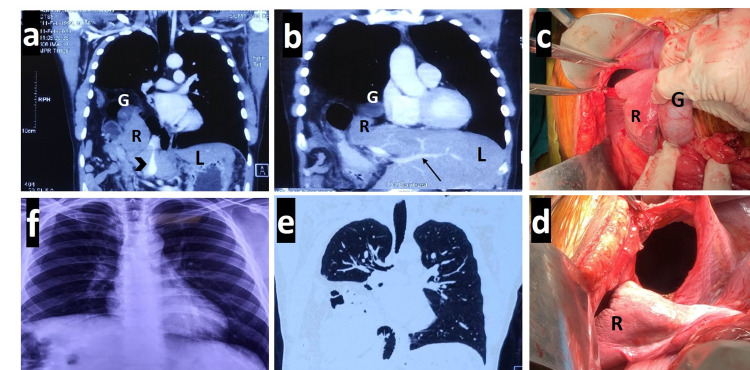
Clockwise from upper left: (a) Coronal contrast CT section of patient 1 showing multiple abdominal viscera entering the right thorax, namely, the colon and small bowel, gallbladder (marked G), and atrophied right liver (marked R). The hypertrophied left liver (marked L) and the middle hepatic vein (marked with black arrowhead) are shown. The right hepatic vein is probably obliterated. (b) Another coronal CT image depicting the complete extent of left liver hypertrophy with the rotated left portal vein axis (marked with black arrow). (c) and (d) Operative images showing the well-defined right posterolateral defect after reduction of contents, with the atrophied right liver (R) and the gallbladder (G). (e) Pre-operative plain CT with multiple viscera in the right hemithorax. (f) Postoperative chest radiograph with complete lung expansion on day seven. CT - computed tomography.
